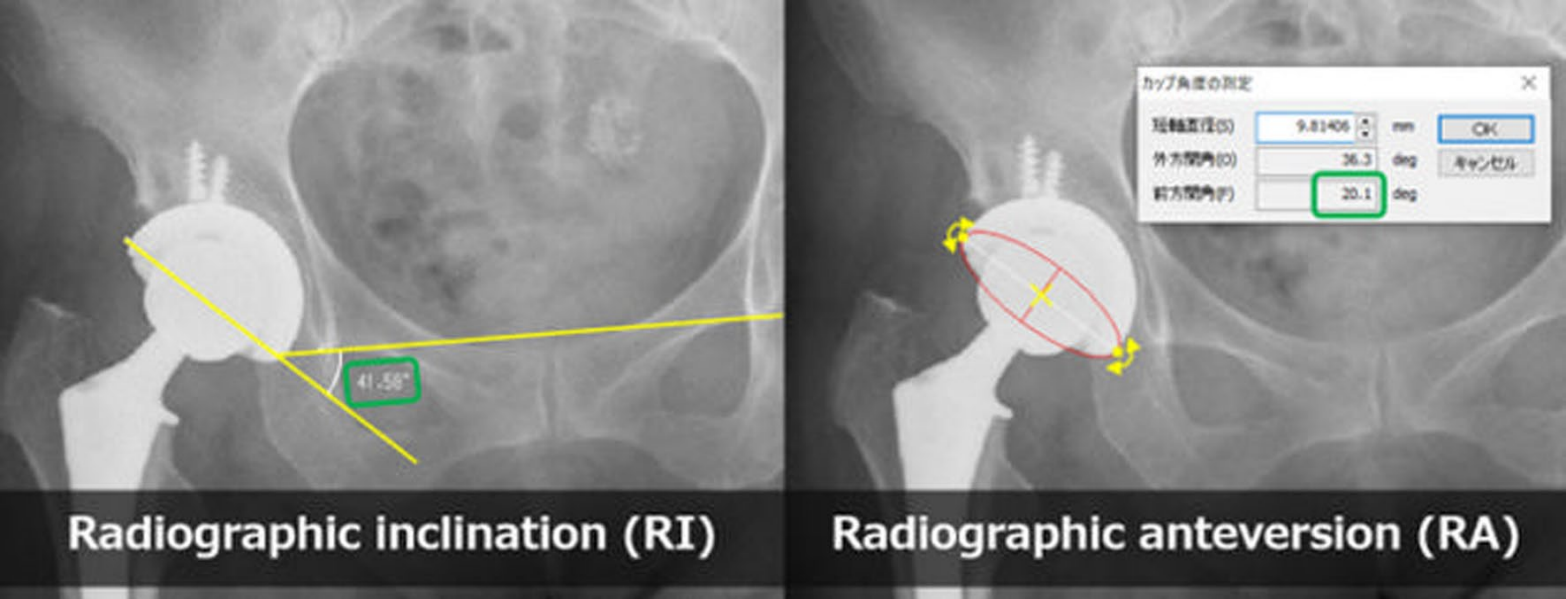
Postoperative radiographic inclination and radiographic anteversion were measured using 2D Template (Kyocera Medical).
